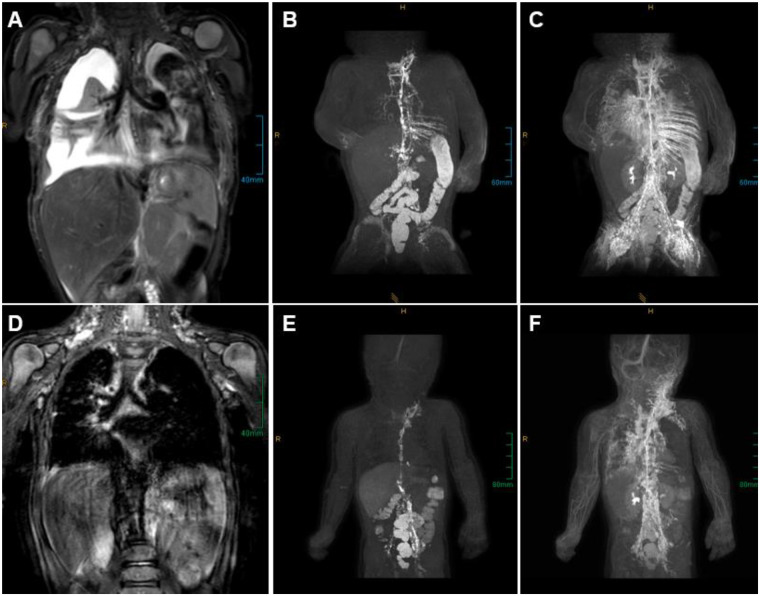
MR-lymphangiography of a 6-month old girl with noonan syndrome (*PTPN11* pathogenic variant) and refractory chylothorax. Coronal T2-weighted mDixon image (water image) demonstrates pleural effusions (A) Coronal maximum-intensity projections (MIP) of a contrast-enhanced MR-lymphangiogram demonstrate thoracic duct dysplasia (with aplasia of the terminal part of the duct) and initial reflux into the left pleura and into peribronchial lymphatics in the early phase (B) and massive reflux into pleural, pulmonary and cervical lymphatics in the late phase (C) After one year of treatment with Trametinib T2-weighted imaging shows improvement of pleural effusions with minimal residual effusions (not visible here) (D) While the early phase of contrast enhancement (E) demonstrates clear improvement of central lymphatic flow with considerably less reflux in comparison to pre-treatment imaging, the late phase (F) shows reduced, but still clear and marked remaining reflux into pleural, pulmonary and cervical lymphatics.
